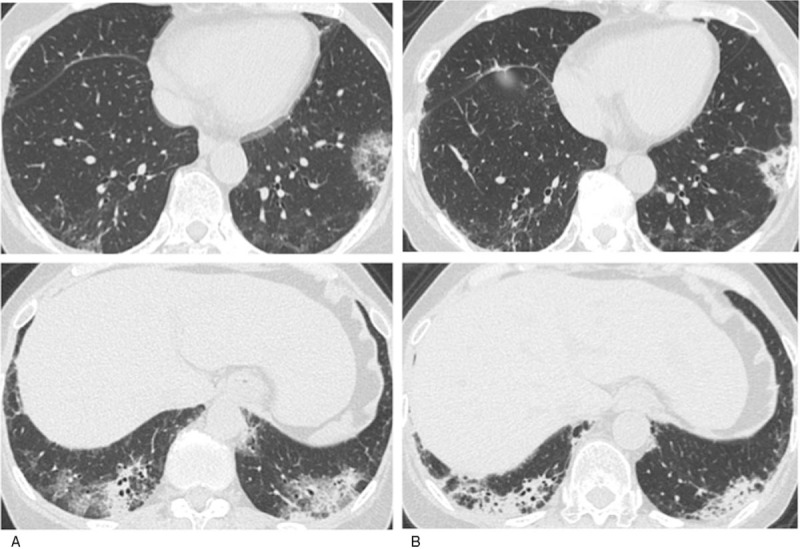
A chest CT on admission showed the expression of invasive shadows on lung field under the pleura and on the dorsal side of the bilateral lower lobes (A), after strengthening treatment it showed contraction of invasive shadows (B).
